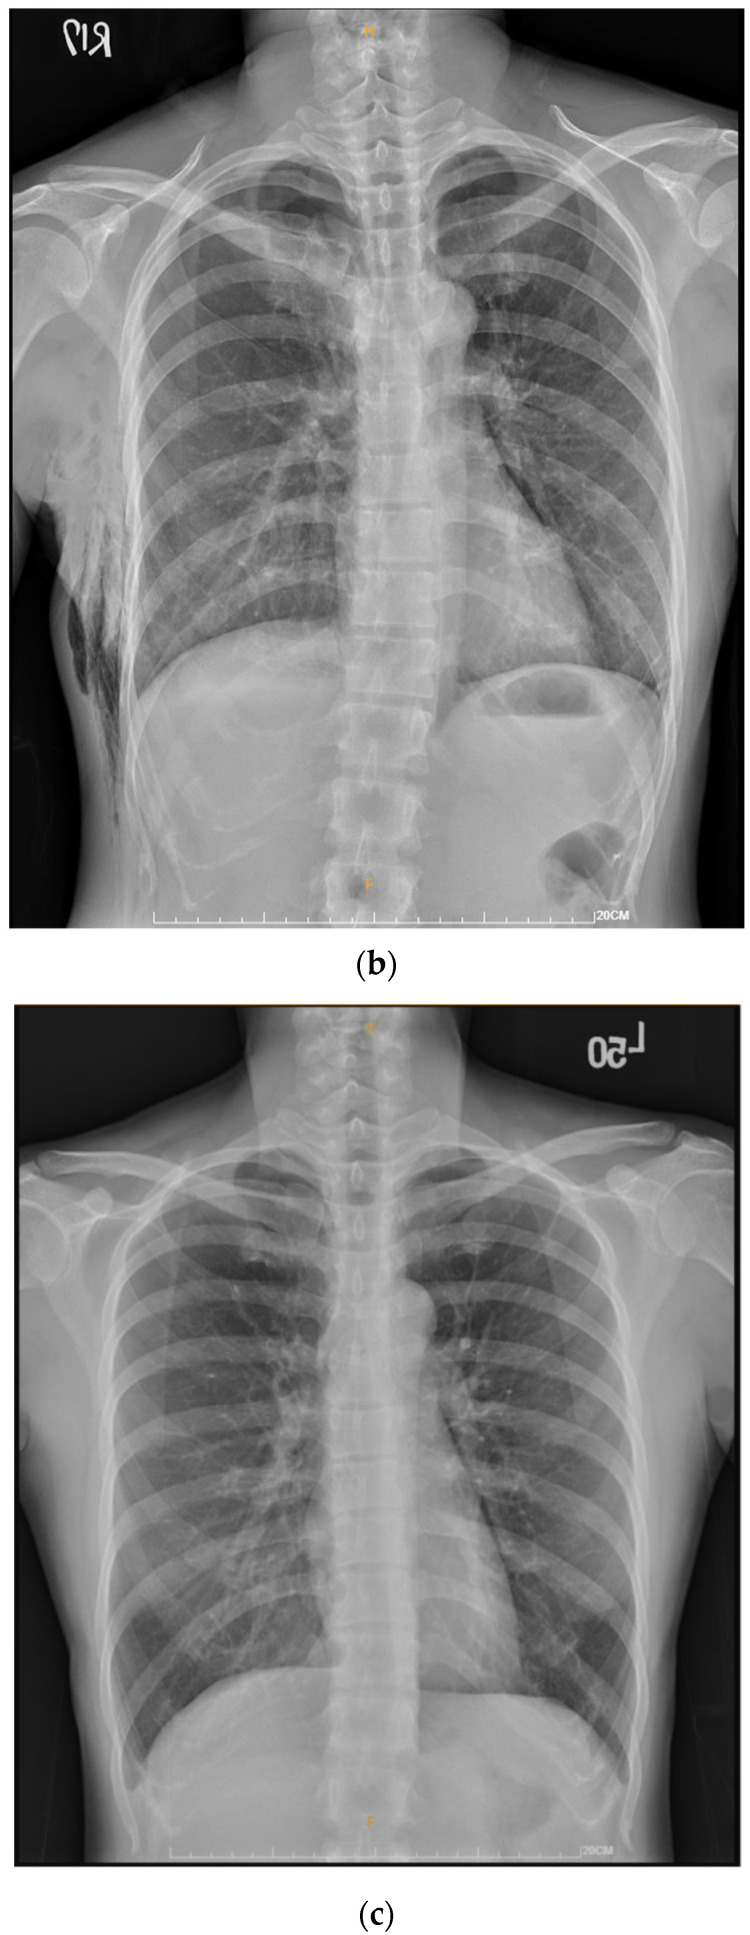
(a) Preoperative CT showed a lung tumor in the right upper lung. (b) The postoperative chest plain film was taken on the operation day immediately after the surgery. Some subcutaneous emphysema was noted on the right side. (c) The chest plain film on the day of the first outpatient return after discharge. No detectable air within the body was noted.
